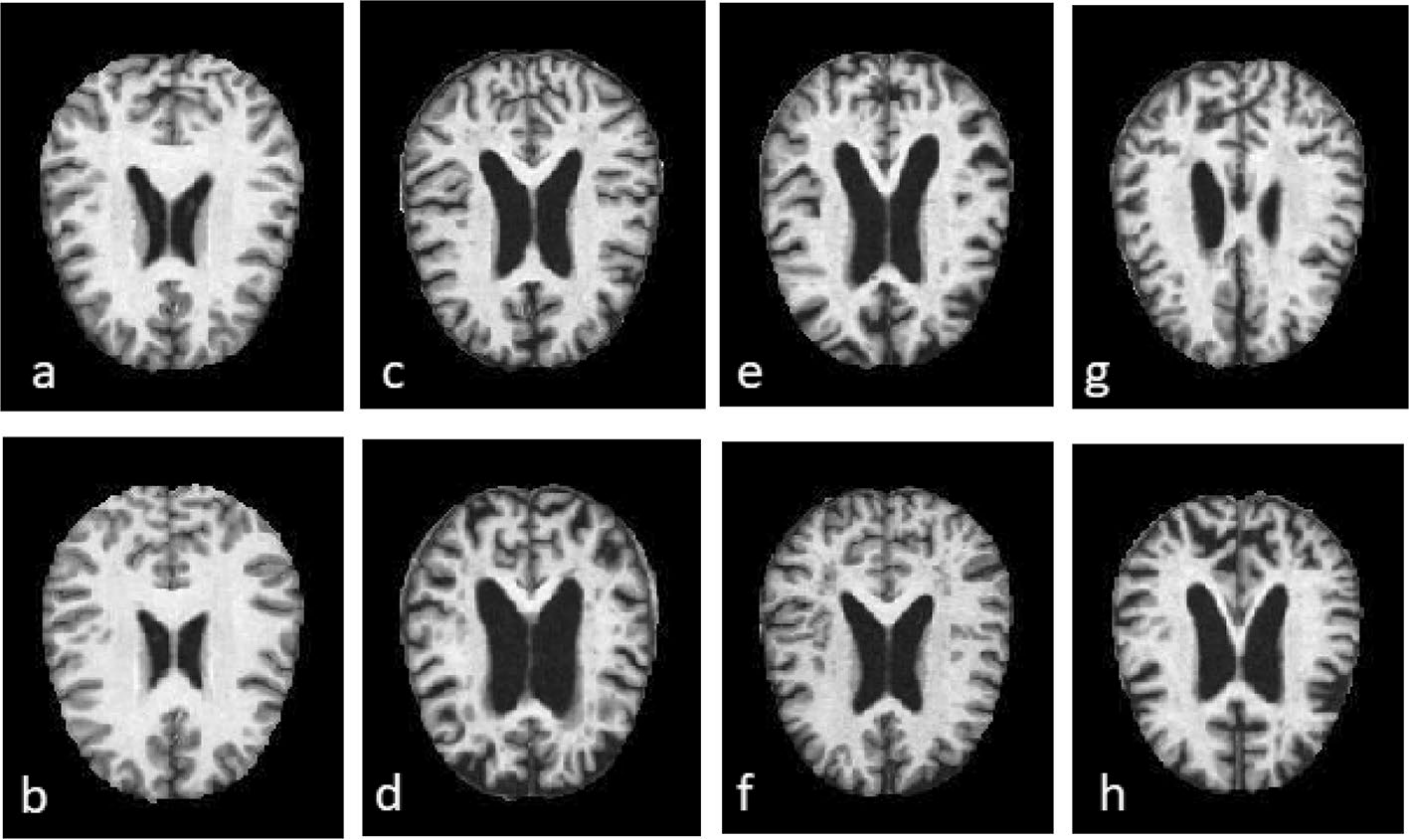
MRI images of dementia cases (a,b) Non-dementia, (c,d) Very mild dementia, (e,f) Mild dementia, and (g,h) Moderate dementia.
